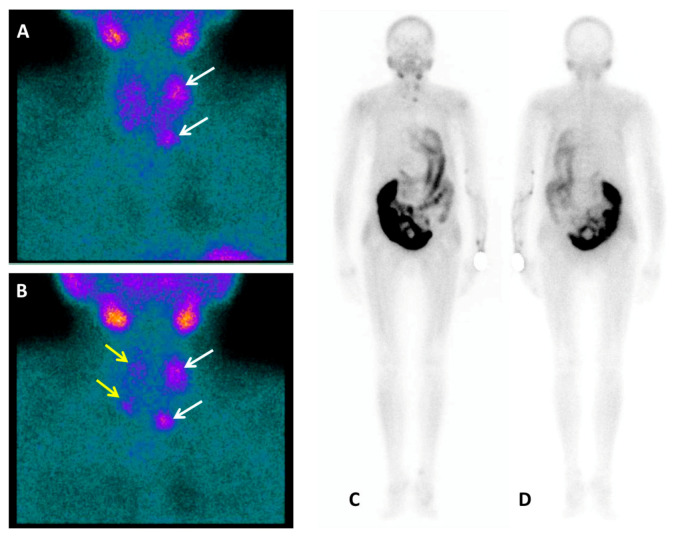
A nephrectomised 62-year-old woman with chronic renal failure (stage 5D, Kidney Disease Improving Global Outcomes (KDIGO)) [1] in dialysis treatment since 2012. Listed for kidney transplantation, laboratory tests were consistent with severe hyperparathyroidism: Parathyroid hormone (PTH) level 2068 pg/mL (normal range 15–57 pg/mL), total calcium level of 7.7 mg/dL (normal range 8.5–10.1 mg/dL), ionized calcium level of 3.49 mg/dL (normal range 4.6–5.3 mg/dL) and inorganic phosphate level of 3.7 mg/dL (normal range 2.5–4.9 mg/dL). Then, patient performed neck ultrasound (US) and, for the recent occurrence of dorsal back pain, a whole-spine magnetic resonance (MRI). According to tertiary hyperparathyroidism (HPT) diagnosis [2] the nuclear medicine imaging workup before surgery consisted of Tc-99m-methoxyisobutylisonitrile (Tc-99m-MIBI) and Tc-99m-methylenediphosphonate (Tc-99m-MDP) planar scintigraphy, completed with Single Photon Emission Computed Tomography/Computed Tomography (SPECT/CT), and ^18^F-FCH PET/CT. Tc-99m-MIBI parathyroid dual-phase planar scintigraphy (anterior view) showed on the early acquisition (A) a left superior hyperfunctioning parathyroid gland together with a left inferior one slightly under the lower third of the left thyroid lobe (white arrows). The delayed acquisition (B) demonstrated, after thyroid gland radiotracer washout, persistent uptake in left parathyroid glands and a mild Tc-99m-MIBI concentration also in the other two right glands (yellow arrows). Planar scintigraphy was integrated with a whole-body acquisition [3] because of the detection of lytic bone lesions on the whole-spine MRI, performed a few days before, doubtful for renal osteodystrophy, giant cell bone tumour, or carcinoma. No Tc-99m-MIBI uptake was found in the bone as showed in the whole body anterior (C) and posterior (D) planar views. Then, patient was directed to Tc-99m-MDP bone scan.
